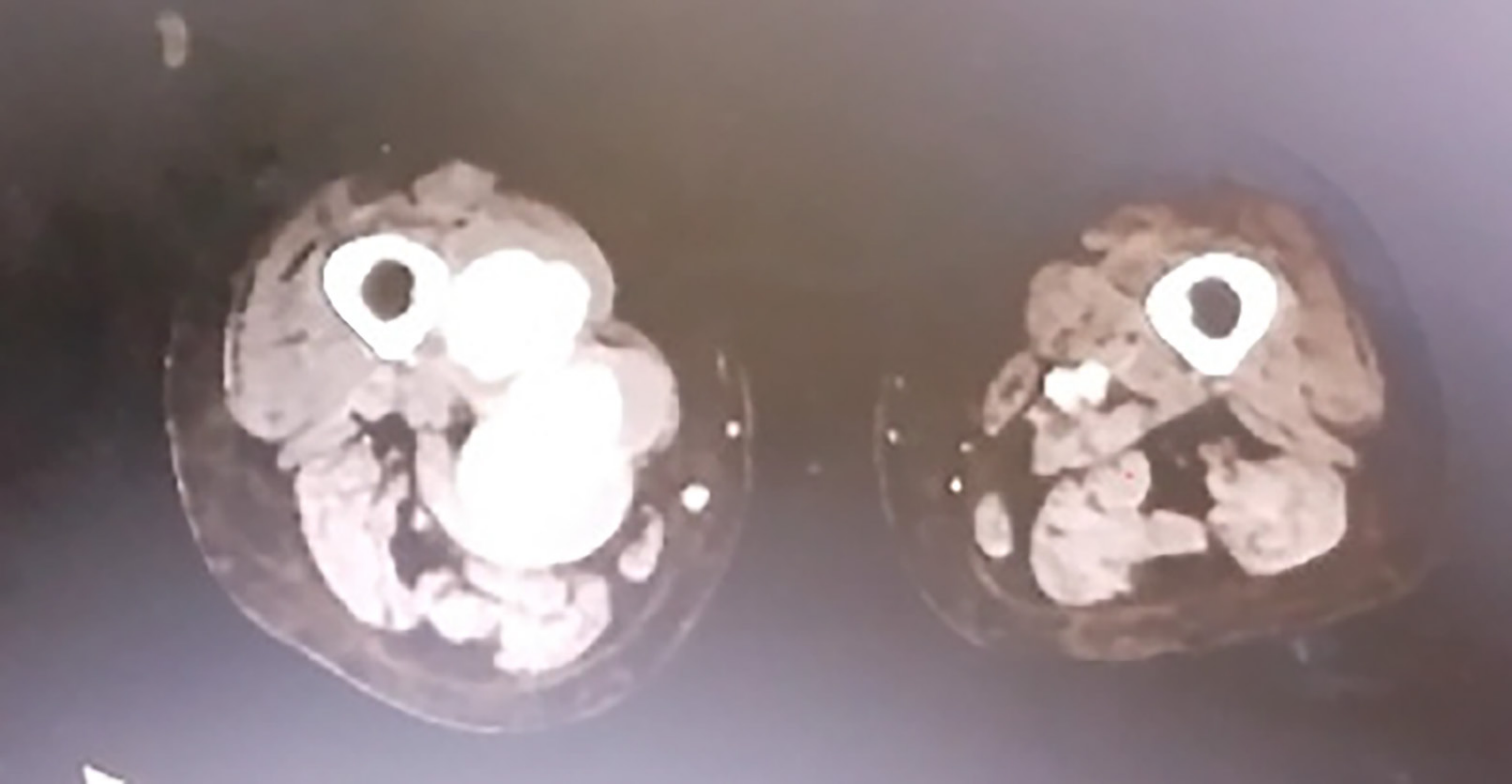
Right thigh CTA showing the aneurysm and AVF.
